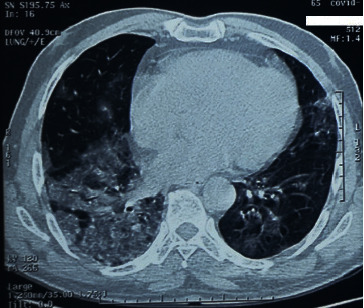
Chest CT scan in the axial section of the mediastinal window showing ground glass images with an asymmetrical peripheral arrangement under the pleural layer, producing a crazy-paving appearance characteristic of typical COVID-19 pneumonia.
